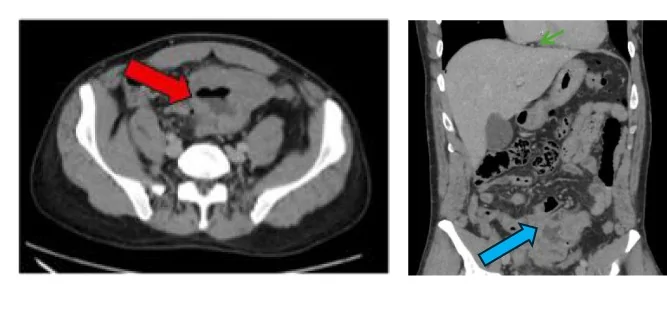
Axial (right) CT of the abdomen and pelvis showing the small bowel with associated abscess 4.5 × 3.3 cm (red arrow). Coronal (left) CT of abdomen and pelvis showing pneumoperitoneum (green arrow) and thickened/distended loops of small bowel with indeterminate mass in the mid abdomen (blue arrow).
